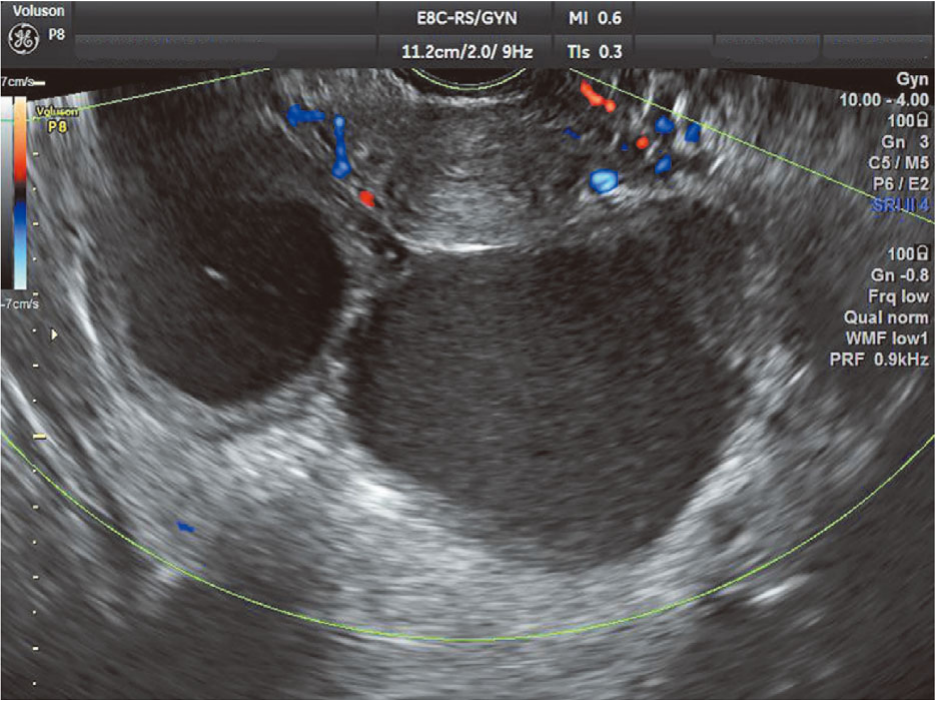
Transvaginal ultrasound coronal plane image. Bilateral ovarian endometrial cysts are localized in the rectovaginal pouch. Shown are cysts adherent to the ovarian tissue and to each other.
